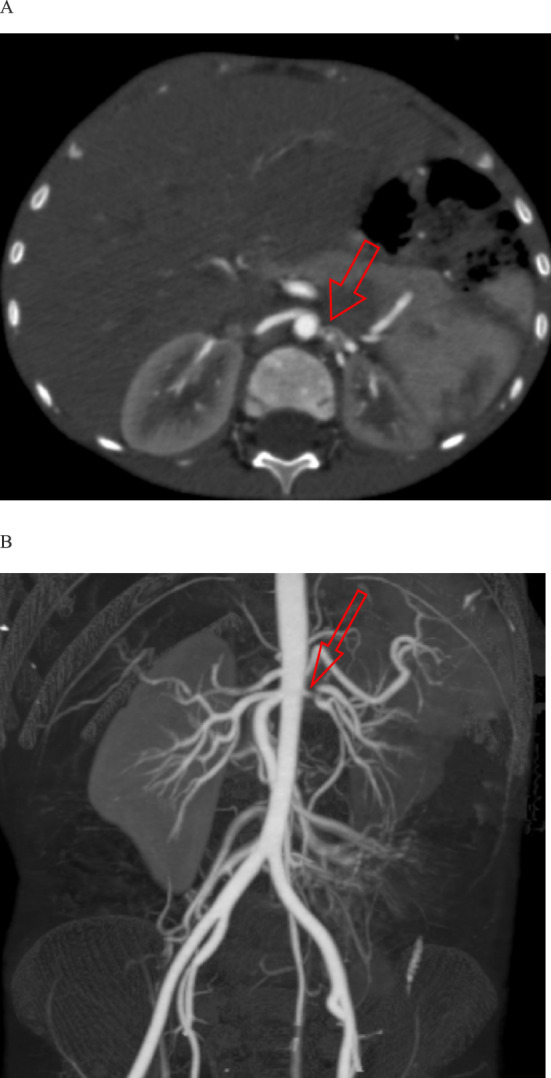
CT angiography (images A, B) showing left renal artery stenosis with small left kidney and enlargement of the right kidney
